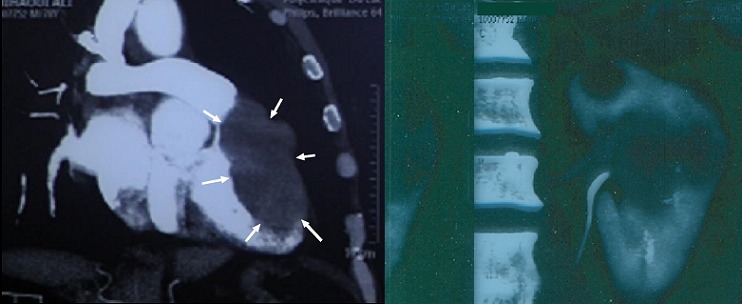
CT scan showing renal tumor and heart tumor
